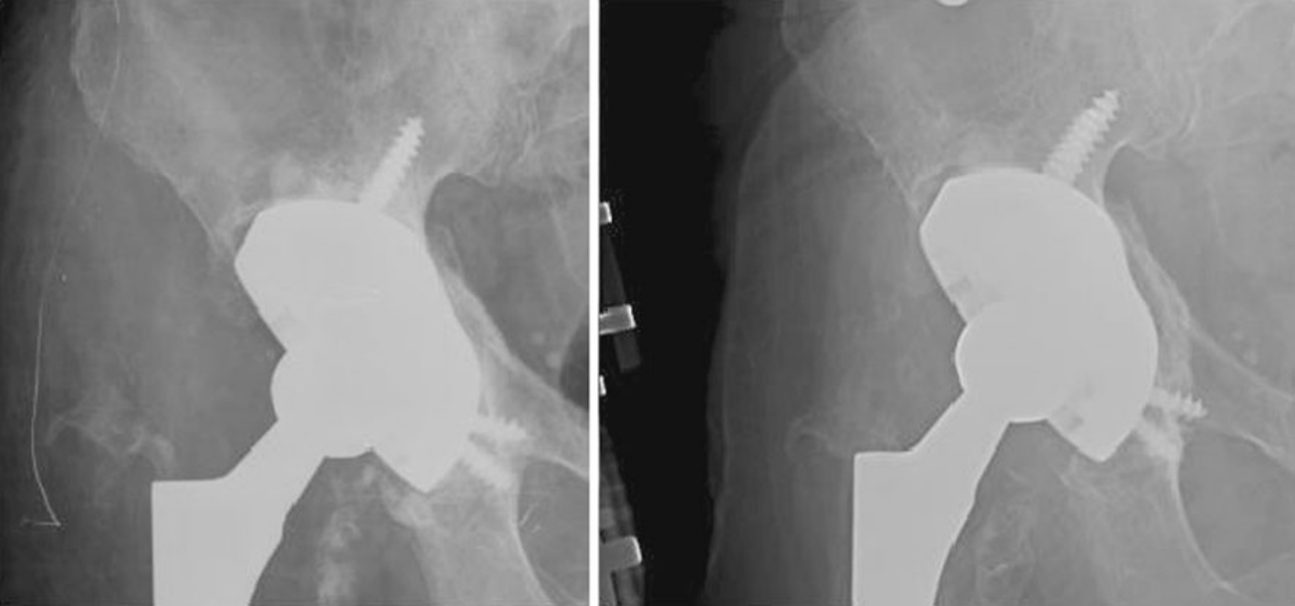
Radiographs of a 75-year-old-man, case 23. a Postoperative radiograph after acetabular reconstruction using a BOFOR cup. b Appearance of a complete radiolucent line and a slight change in the position of the cup at four years (the patient used a cane and related mild groin pain)
